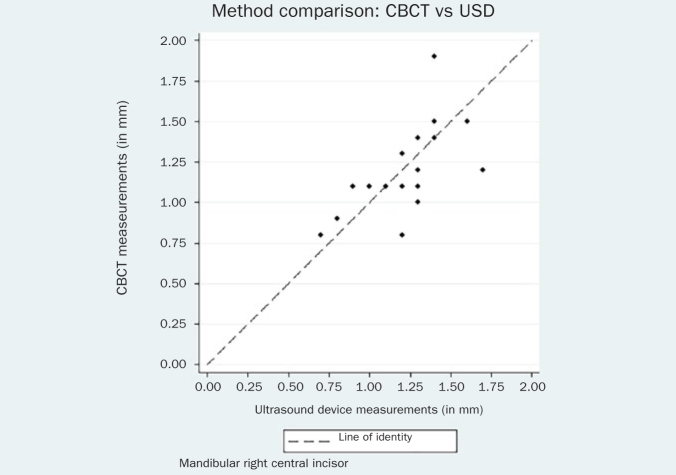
Method agreement plot CBCT vs USD 41.
